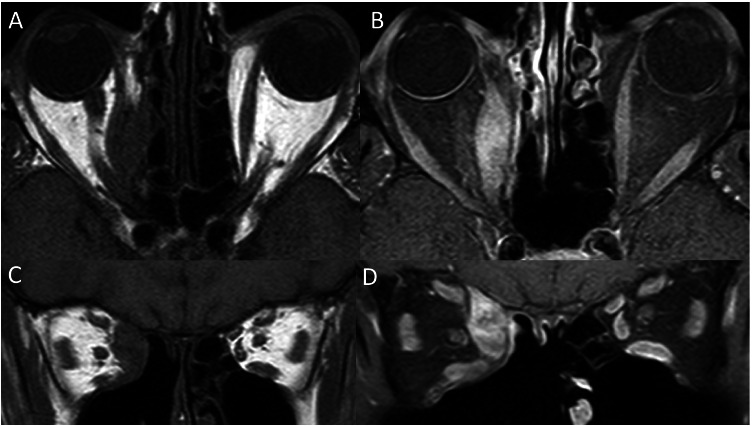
Granulomatosis with polyangiitis (Wegener’s). Axial T1-weighted MRI (A) and fat-suppressed contrast-enhanced T1-weighted MRI (B) showing fusiform enlargement of the right medial rectus muscle with anterior tendon sparing. Coronal T1-weighted MRI (C) demonstrates isointense enlargement of the right medial rectus. Coronal fat-suppressed contrast-enhanced T1-weighted MRI (D) shows heterogenous enhancement and enlargement of the right medial rectus
